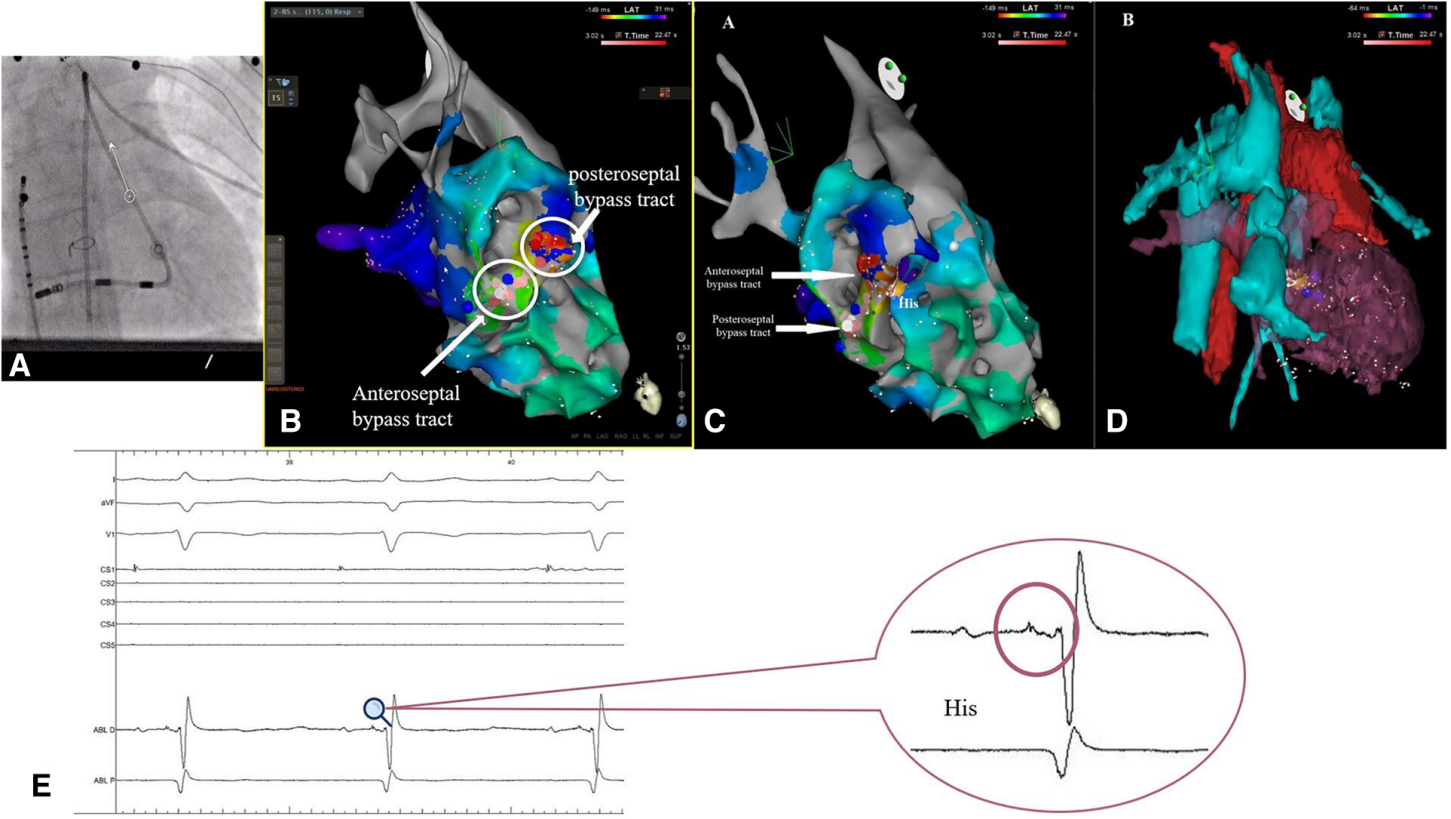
(A) Fluoroscopic imagine showing the position of the decapolar deflectable catheter into the intra-atrial conduct, with no puncture of the dacron conduit and the position of the thermocool RMT catheter (B,C) electroanatomical CARTO 3 map showing the position of the two accessory pathways in relation to the His bundle (yellow dot). (D) Electroanatomical reconstruction using computed tomography. (E) Intraprocedural electrogram of the His bundle.
